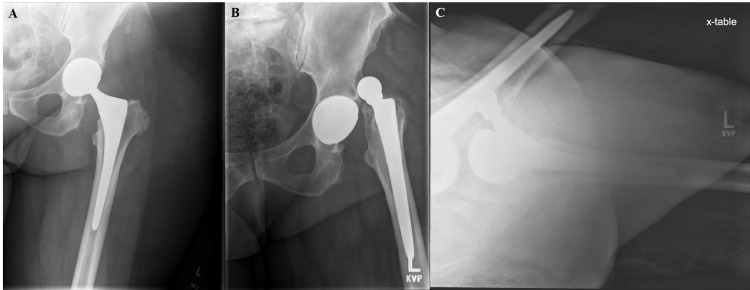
Anterior-posterior hip radiograph (A) at two weeks postoperatively after undergoing left THA (Stryker Trident, modular dual mobility, high offset stem) using a modified Hardinge approach. Patient sustained a fall and dislocated anteriorly as seen on anterior-posterior (B) and cross table lateral (C) radiograph.
